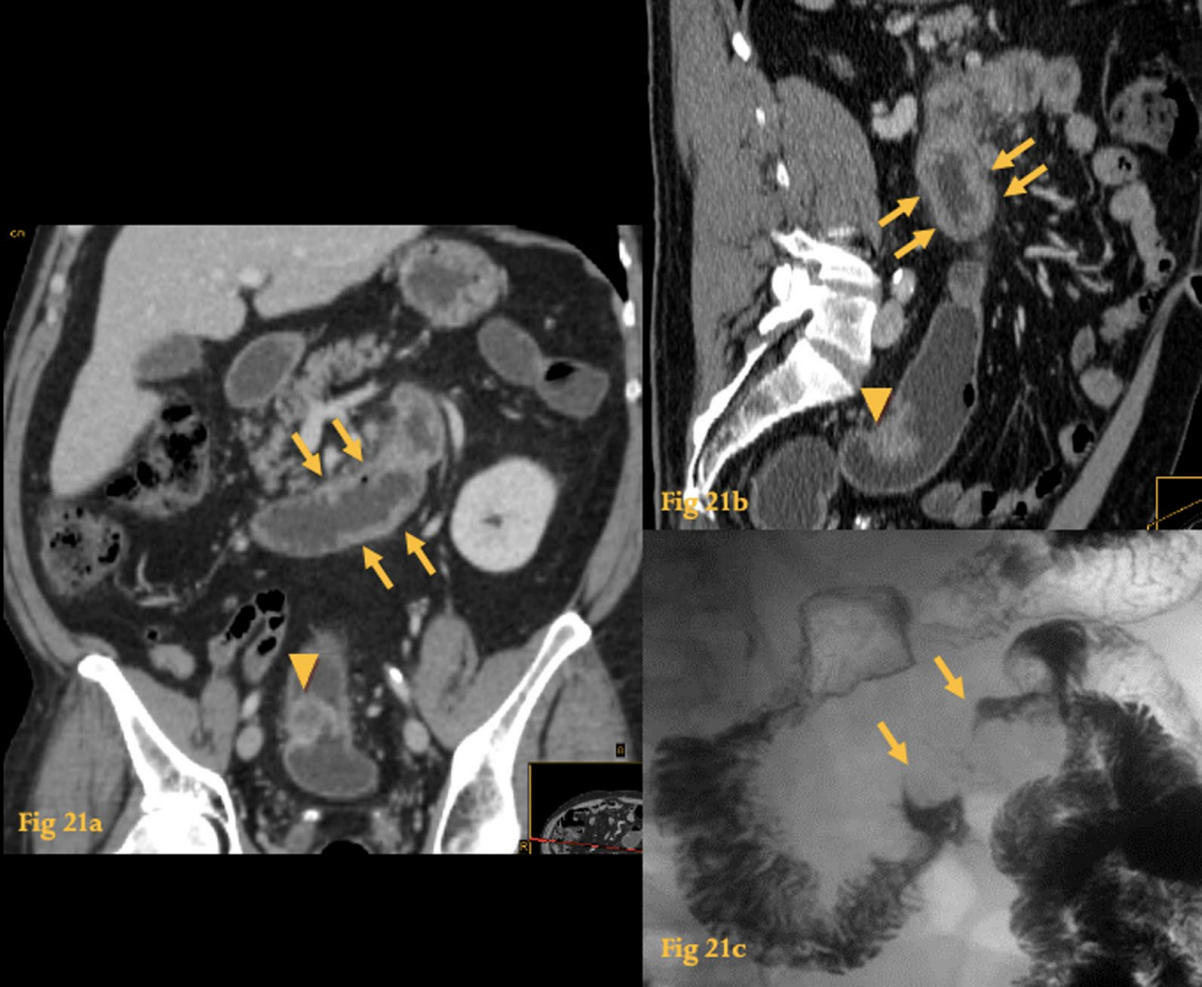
Metastasis. Abdominal and pelvic CT (a, b) of a patient with sigmoid colon cancer, performed to stage the disease, clearly demonstrated the sigmoid tumor (arrowheads) but failed to show distant metastases. However, MPR and retrospective analysis revealed a discrete circumferential thickening on D3 and D4 (arrows) which was not recognized initially. This duodenal lesion progressed to become an “apple core” lesion (arrows) causing obstructive symptoms, as evaluated by a barium study performed 2 months later (c)
